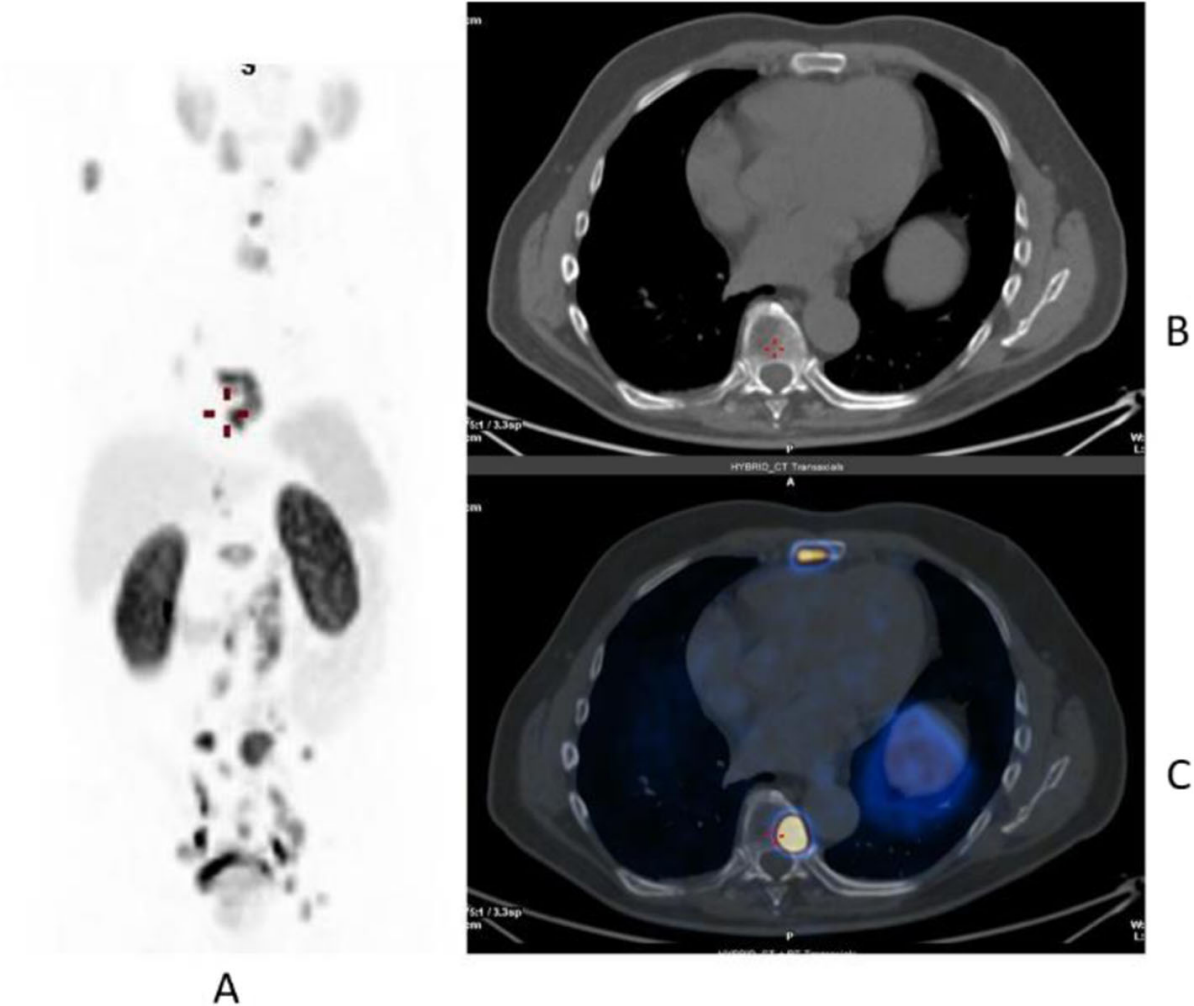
^68^Ga-PSMA, prostate cancer, staging. *Clinical history*: 56 y.o. man with prostate cancer. At presentation, Gleason score 4 + 5; PSA = 14 ng/ml, candidate to radical prostatectomy. *PET/CT findings*: multiple foci of increased uptake involving prostate, lymph nodes, and bones (a MIP). Vertebral lesion seen on fused images (c) is not evident on CT (b)
